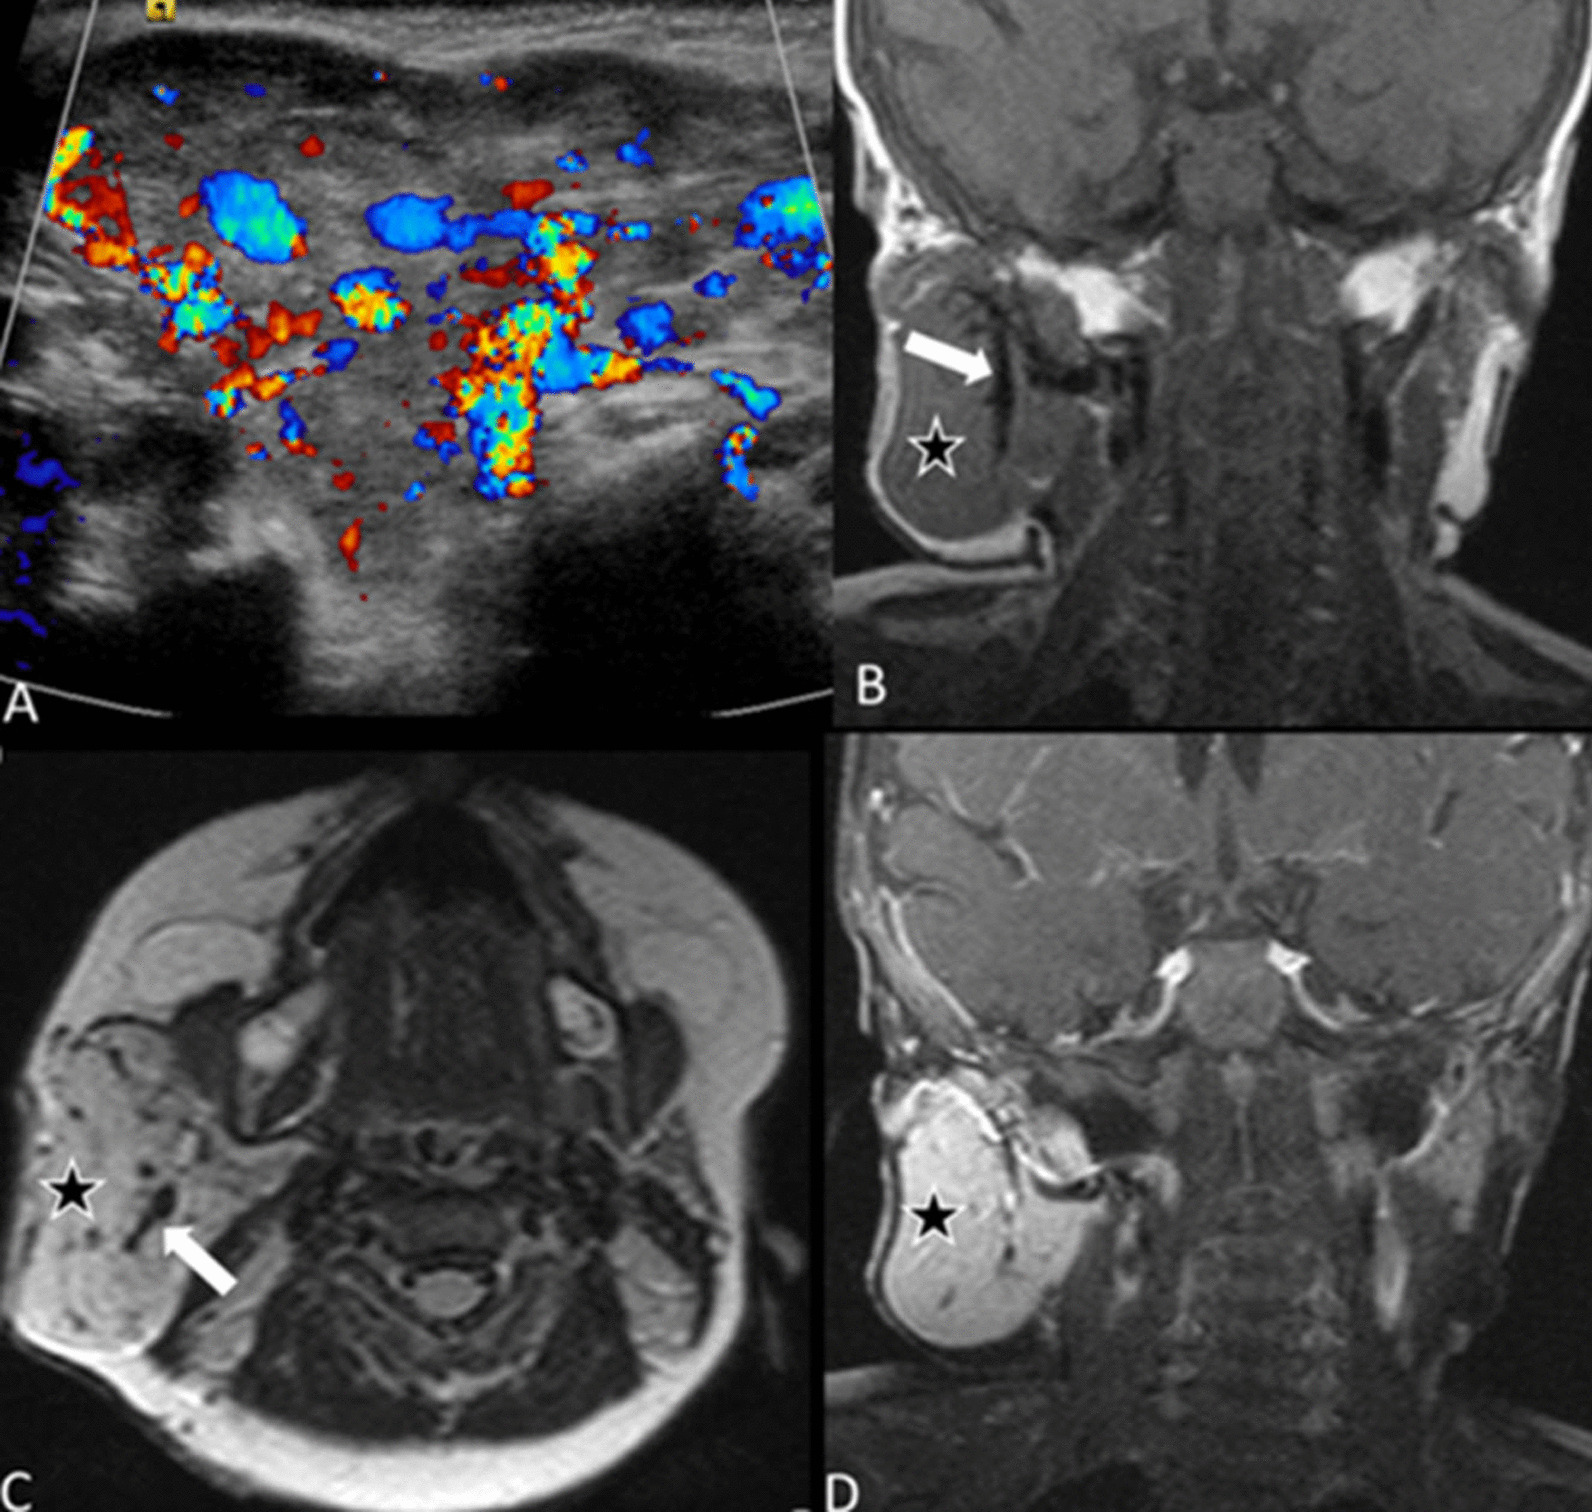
Infantile hemangioma. A 4-month-old female child with a hemangioma (star) on the right side, present since birth, with a marked increase in the size over the previous 2 months. Doppler ultrasound (A) shows the characteristic vascular nature of the mass. The lesion is hypointense on T1-w (B), hyperintense on T2-w (C) with diffuse homogeneous enhancement (D). Note the prominent vessels with flow voids in the lesion (white arrows)
